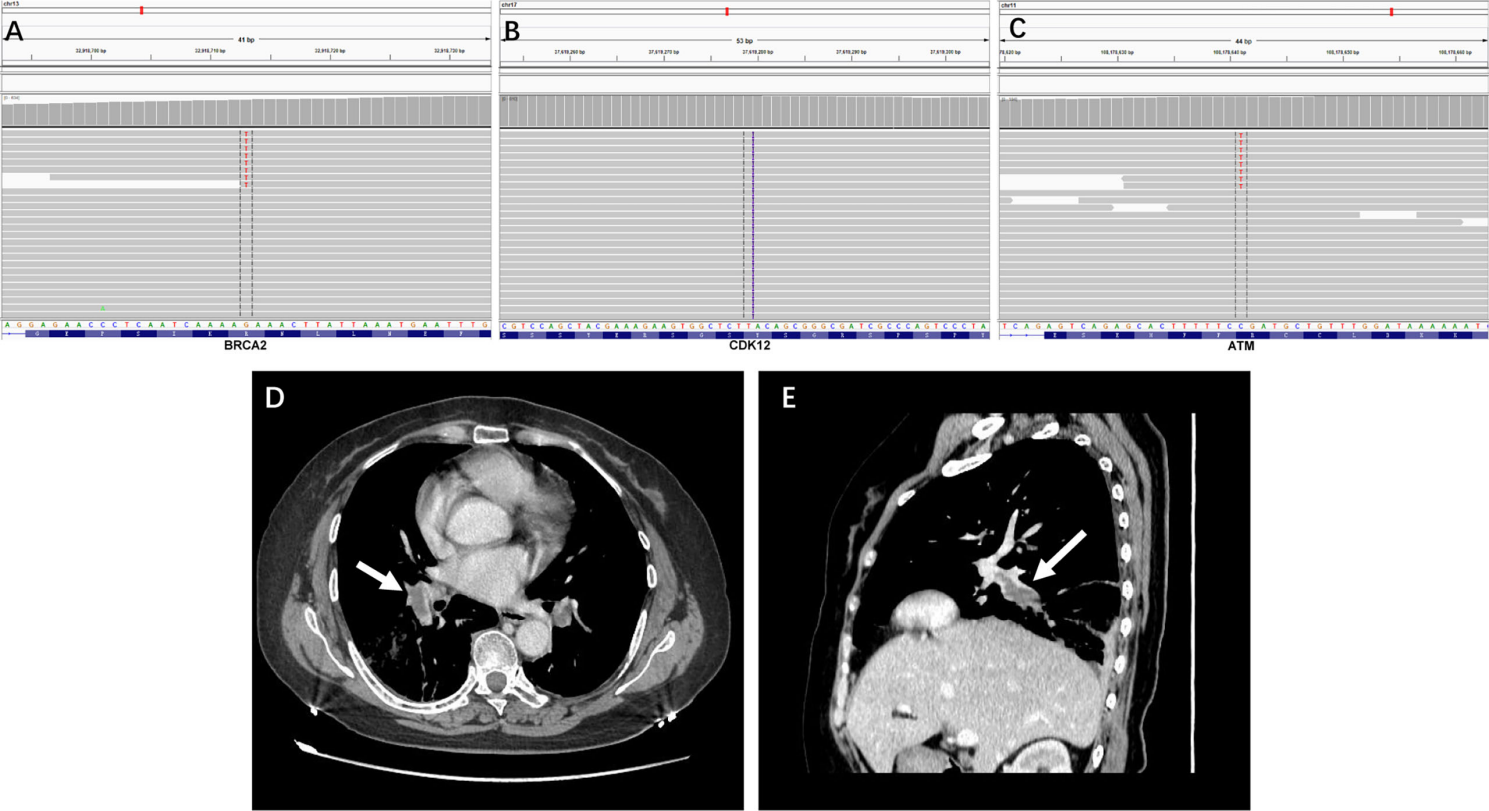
(A–C) The integrative genomics viewer snapshot of *BRCA2* c.6860G>T, *CDK12* c.956dupA, and *ATM* c.5692C>T mutation. (D, E) Contrast-enhanced CT revealed dilated pulmonary veins with hypodense filling defect (E), 35.0 × 13.2 mm) highlighting the presence of thrombosis (arrow).
